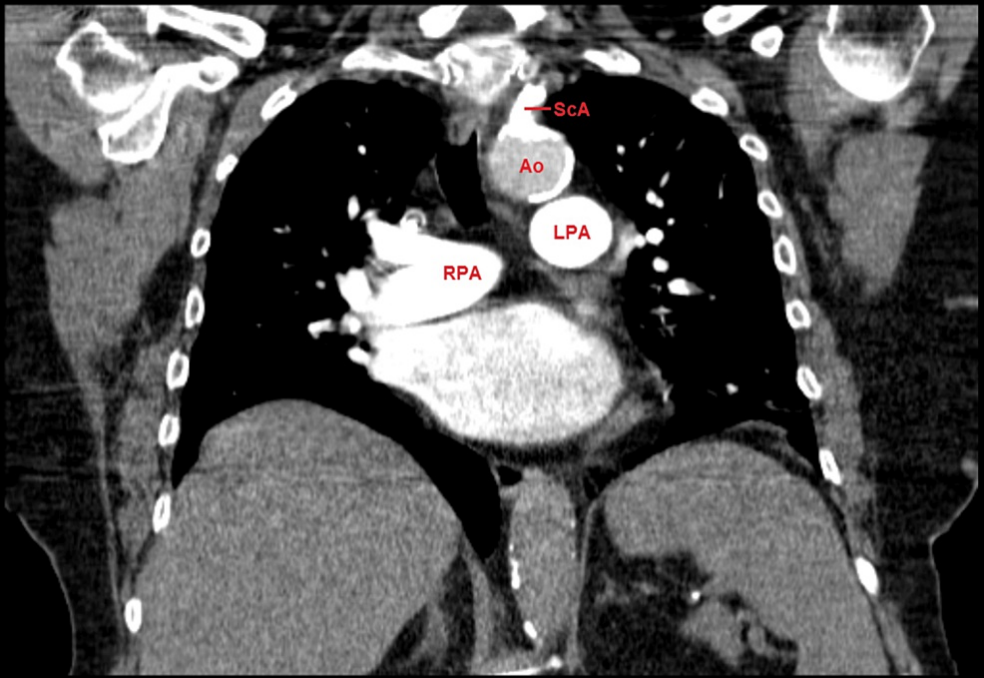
CT scan angiography of the supra-aortic arch: calcification visible at the emergence of the left subclavian artery. ScA: left subclavian artery; Ao: aorta; RPA: right pulmonary artery; LPA: left pulmonary artery
